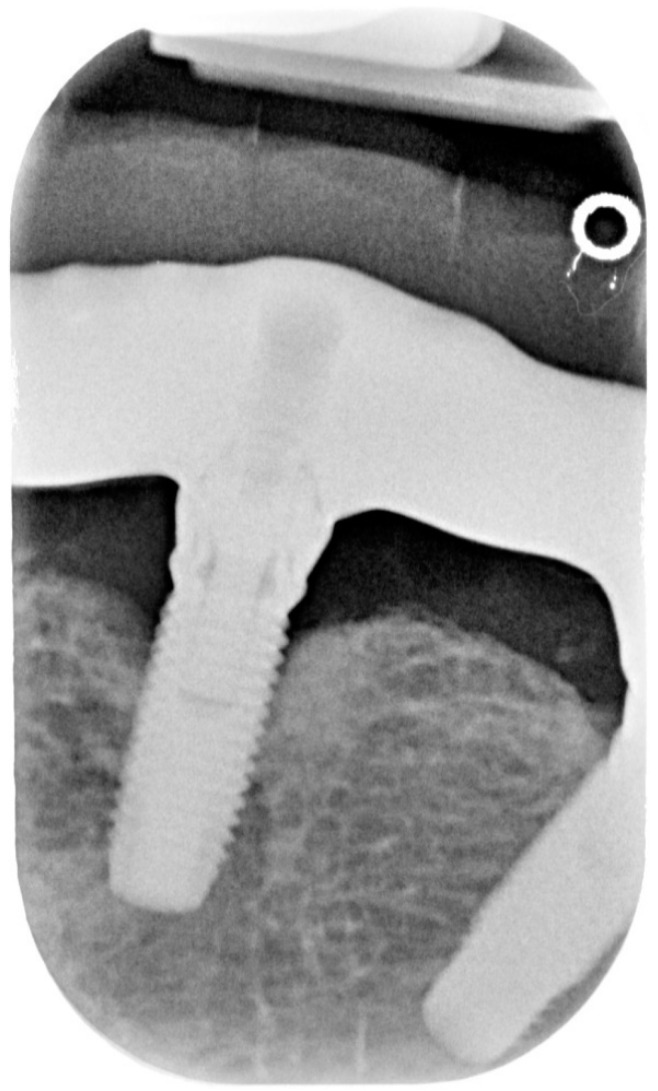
X-ray of dental implant area before treatment.
